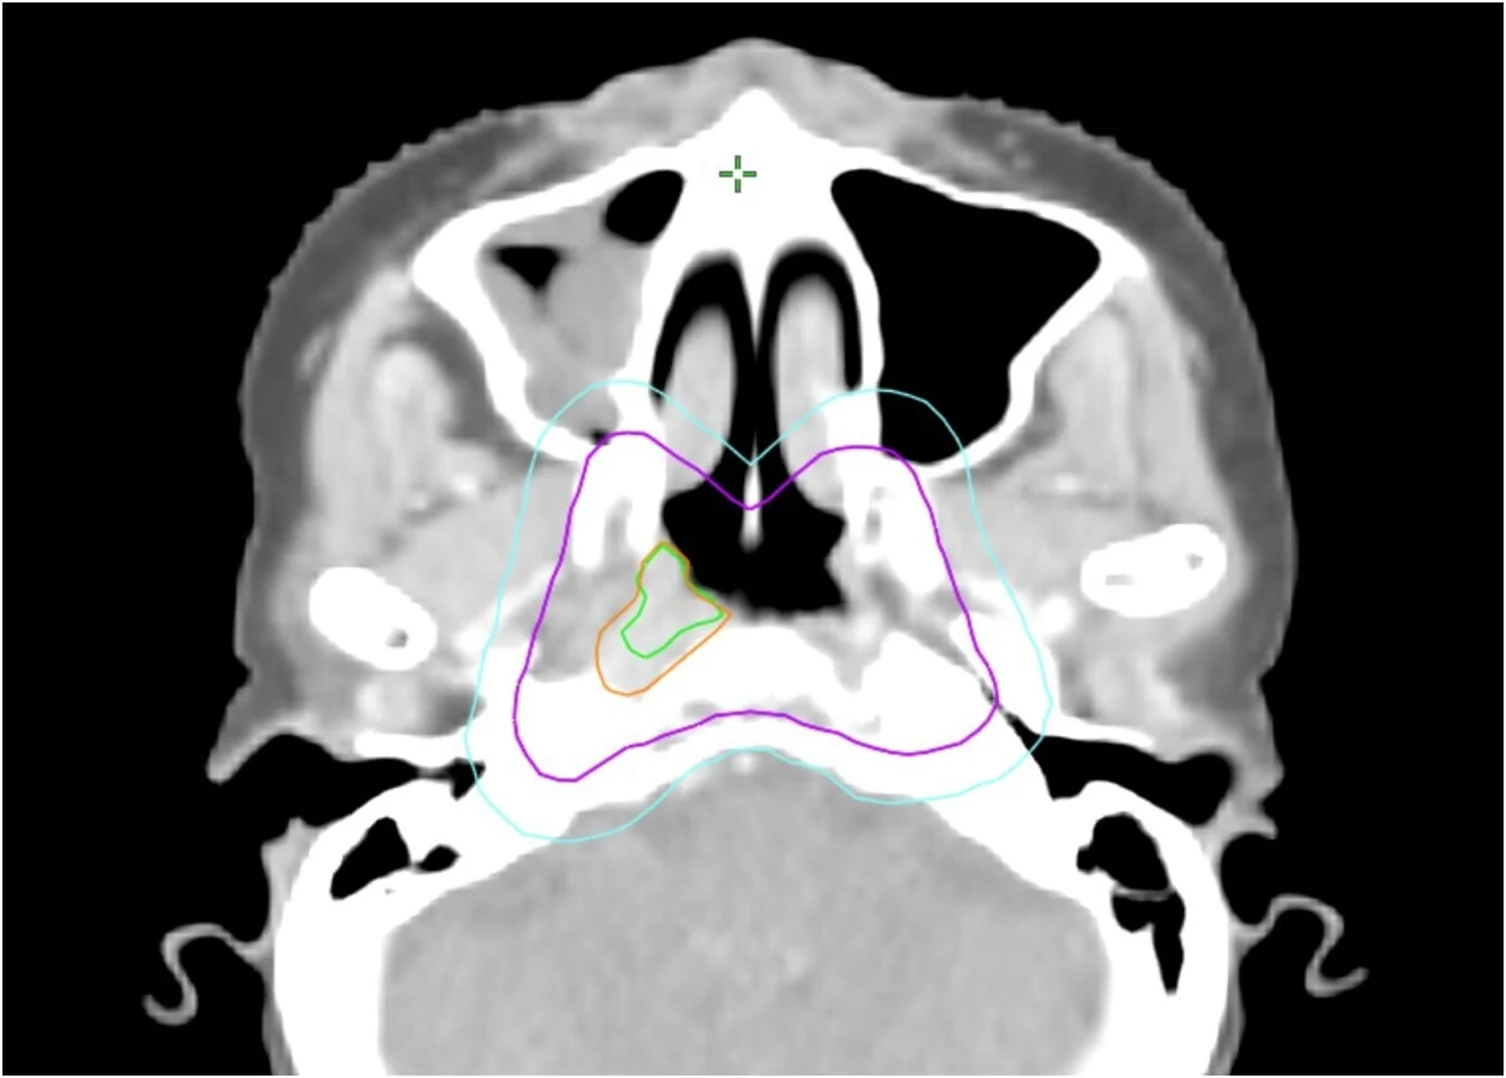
Target volume segmentation for a representative patient. Axial CT image shows one observer's segmentation of GTVnx (GTVnx_OS: brown), reference segmentation of GTVnx (GTVnx_ref: green), CTV (red: original, blue: adjusted) and PTV (light blue). Original CTV and adjusted CTV are perfectly overlapped (purple) due to high anatomical accuracy.
